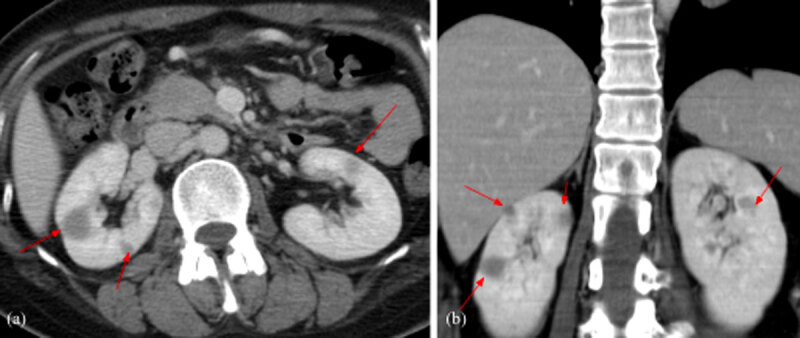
Contrast-enhanced abdominal CT scan axial (a) and coronal (b) views of the kidneys: Multiple poor enhancing low attenuation lesions in the bilateral kidneys.
